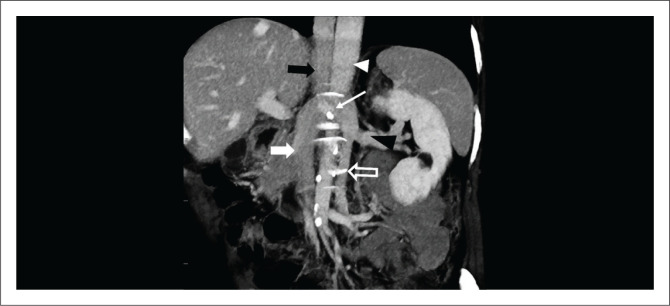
Coronal oblique CT reconstruction of a contrast enhanced abdomen shows a double inferior vena cava (IVC) with left and right IVCs indicated by an open white arrow and a solid white arrow, respectively. The IVC then continues as the azygos vein (black thick arrow) to the right of the aorta (white arrowhead). The left renal vein (black arrowhead) courses posterior to the aorta to the right side to drain into the right IVC as shown by thin white arrow.
